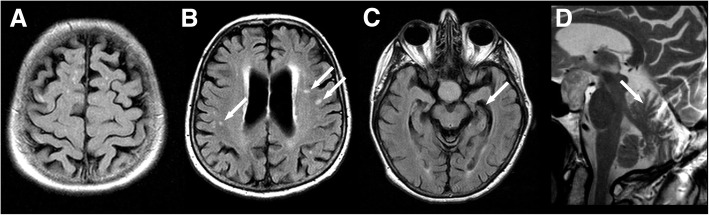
Cerebral MRI six months after symptom onset showed cortical atrophy (a), multiple, mainly periventricular white matter lesions (b), bilateral mesiotemporal atrophy (c) and cerebellar atrophy (d). The findings are not characteristic for ALS, but occur regularly in patients with GABA_B_ receptor encephalitis
